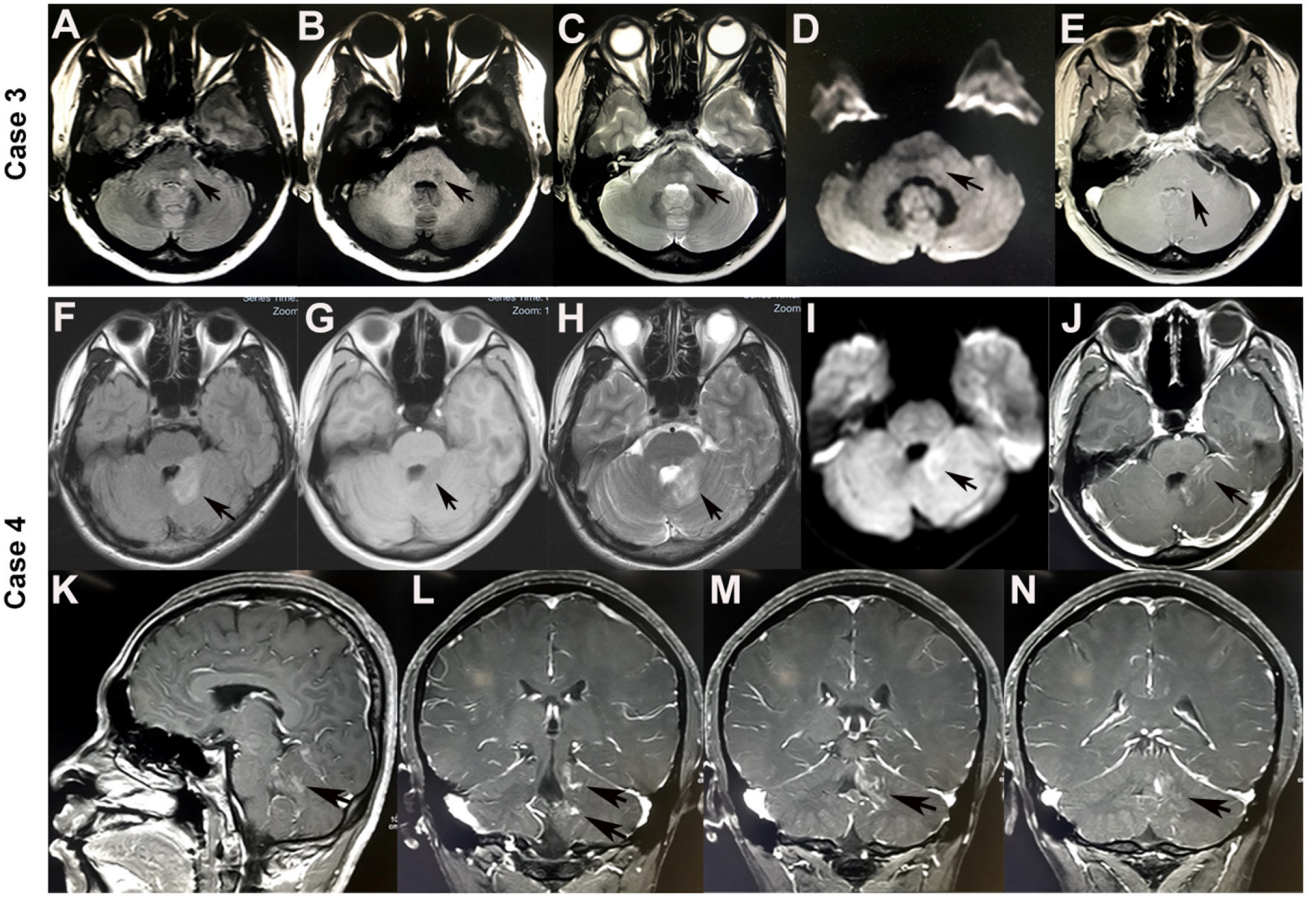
Brain MRI of brainstem lesions in cases 3 and 4. A nodular hyperintense lesion in the left brachium pontis on FLAIR (A), T2-weighted (C), and diffusion-weighted (D) images (arrowheads). However, it was hypointense on the T1-weighted image (B), and with a gadolinium-enhanced edge on the T1-weighted image (E) (arrowheads). Hyperintense lesions in the left brachium pontis, left cerebellar hemisphere, and cerebellar vermis on FLAIR (F), T2-weighted (H), and diffusion-weighted (I) images (arrowheads). However, it was hypointense on the T1-weighted image (G), and with curvilinear post-gadolinium contrast enhancement on the T1-weighted image: axial (J), sagittal (K), and coronal (L–N) (arrowheads).
